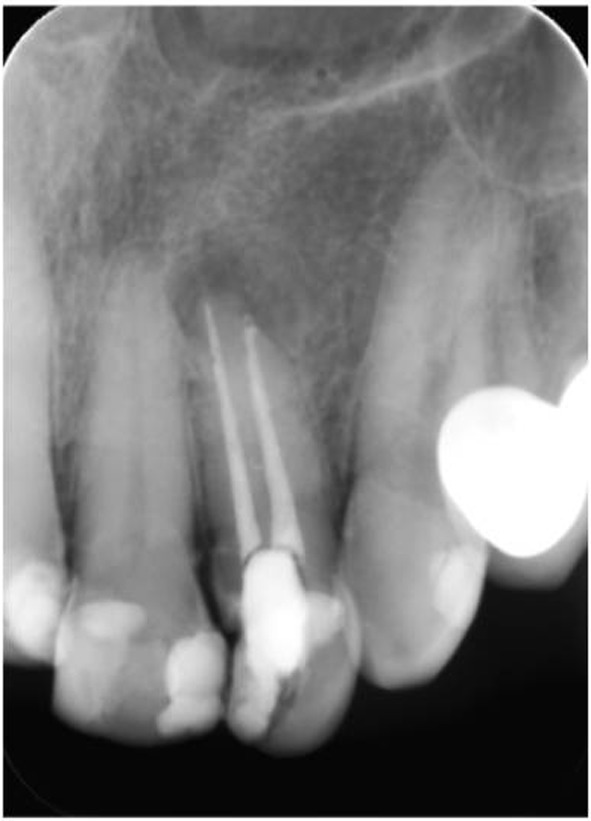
Postoperative periapical radiography.
